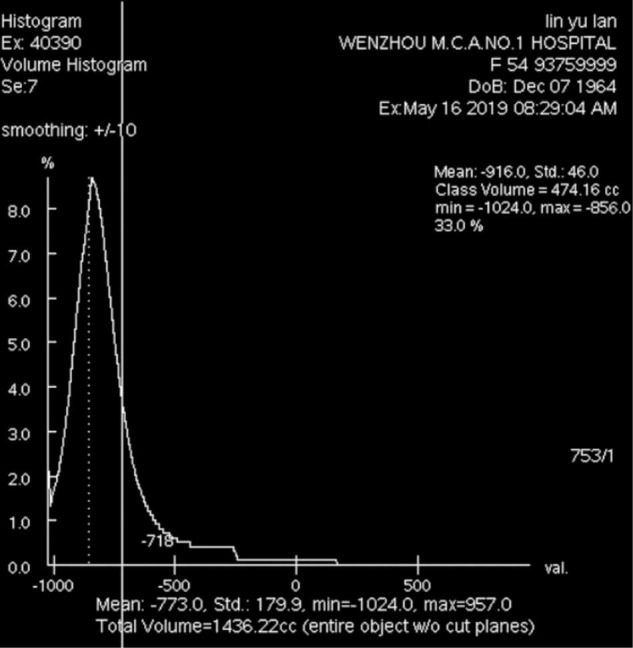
Air trapping volume, total lung volume, air trapping index and MLDexp of the entire lung on expiratory CT scan (–856 HUs).
